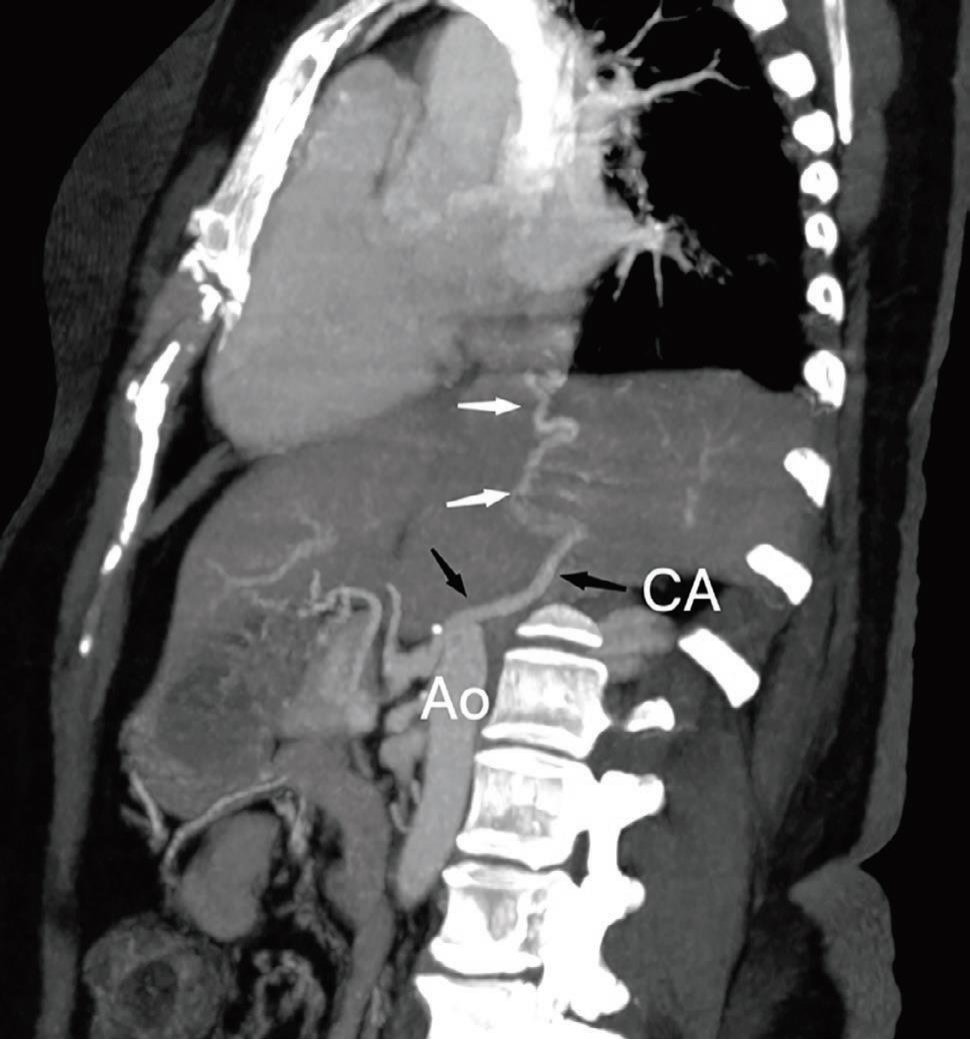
CT angiogram phase in curved multiplanar reconstruction and maximum intensity projection in sagittal view showing subdiaphragmatic collateral branch (white arrows) from celiac artery (CA) (black arrows) supplying the right lower lung.
